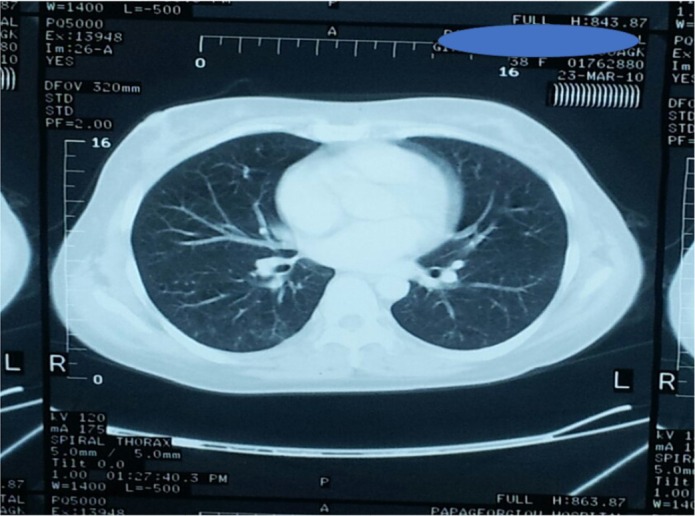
High resolution chest CT scan showing improvement of the lung disease.
